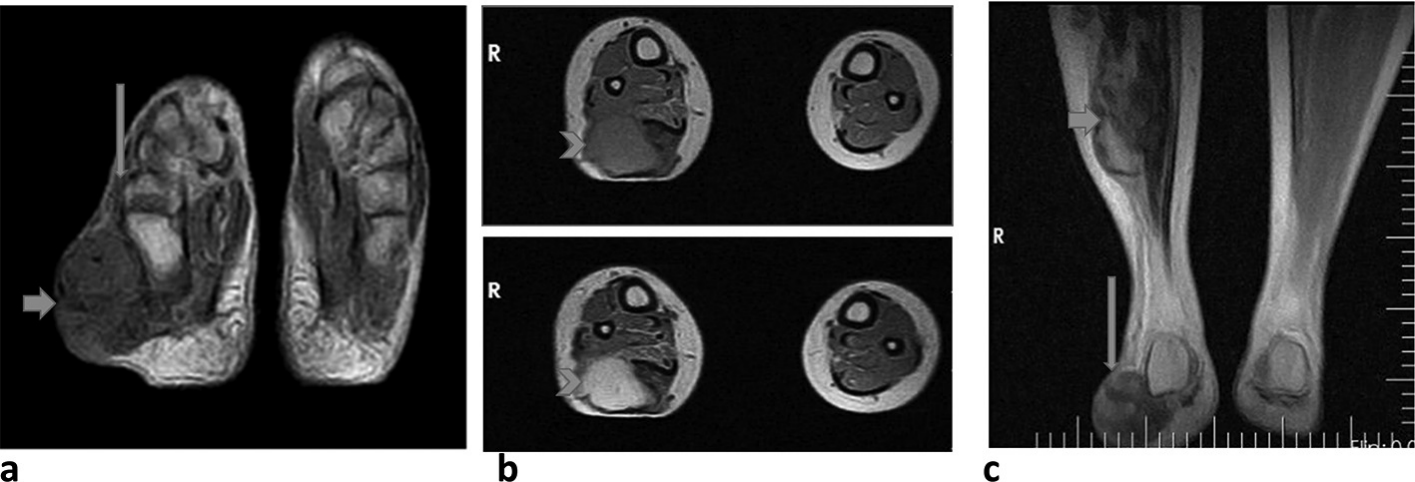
(a) Pre-contrast spin echo axial *T*_1_ weighted MR image (TR/TE, 904/16) shows a bulging mass (short arrow) in the subcutaneous tissue over the right ankle which is iso-intense to adjacent muscle (long arrow). (b) Pre- and post- contrast spin echo axial *T*_1_ weighted MR images (TR/TE, 904/16) show enhancement of the right posterolateral calf mass (arrow heads). (c) Coronal *T*_2_ weighted fat-saturated MR image (TR/TE, 2000/32) shows a bulging mass (short arrow) in the subcutaneous layer of the right calf and right ankle (long arrow) which is hyper-intense to adjacent muscle.
